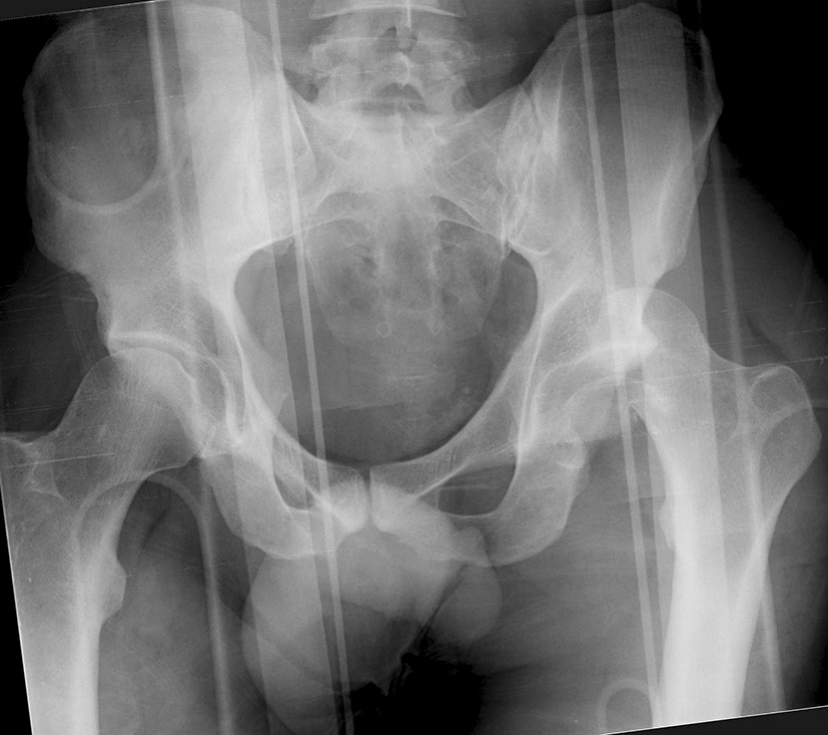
Anteroposterior (AP) pelvis radiograph of a 36-year-old male involved in a motor vehicle collision with a left femoral head fracture dislocation
